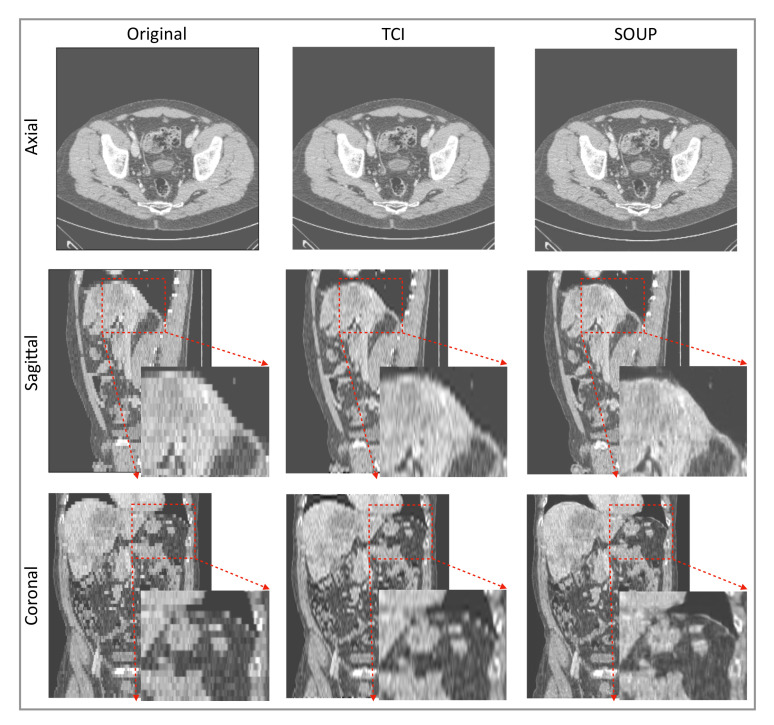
Another example of SR interpolation on CT data with a sampling factor equal to 5.72. TCI: tricubic interpolation.
